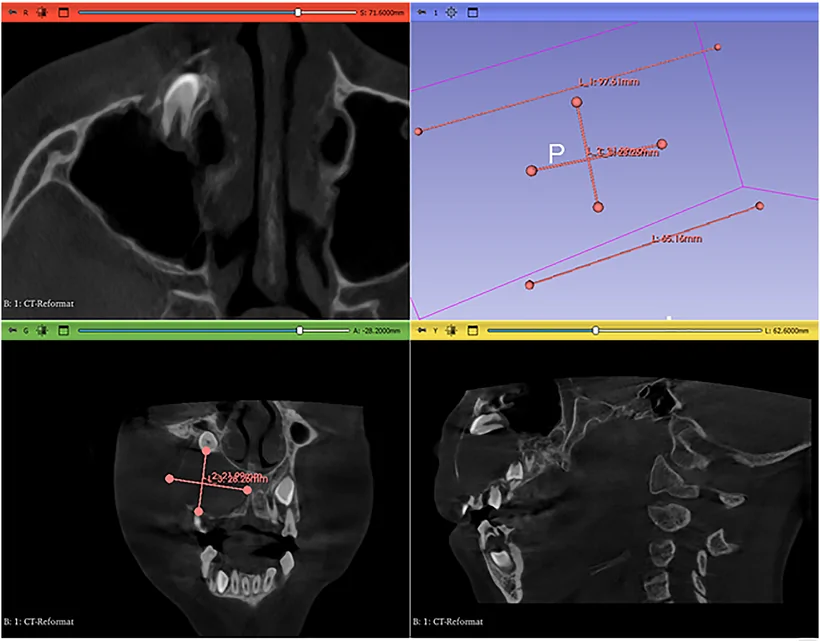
CBCT reconstruction showing the maxillary cyst.
